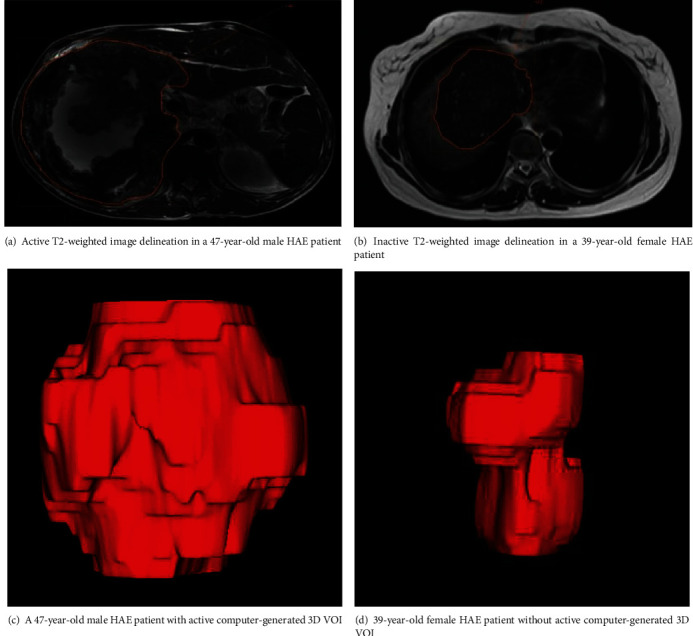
A representation of the manual segmentation in the T2-weighted images.
